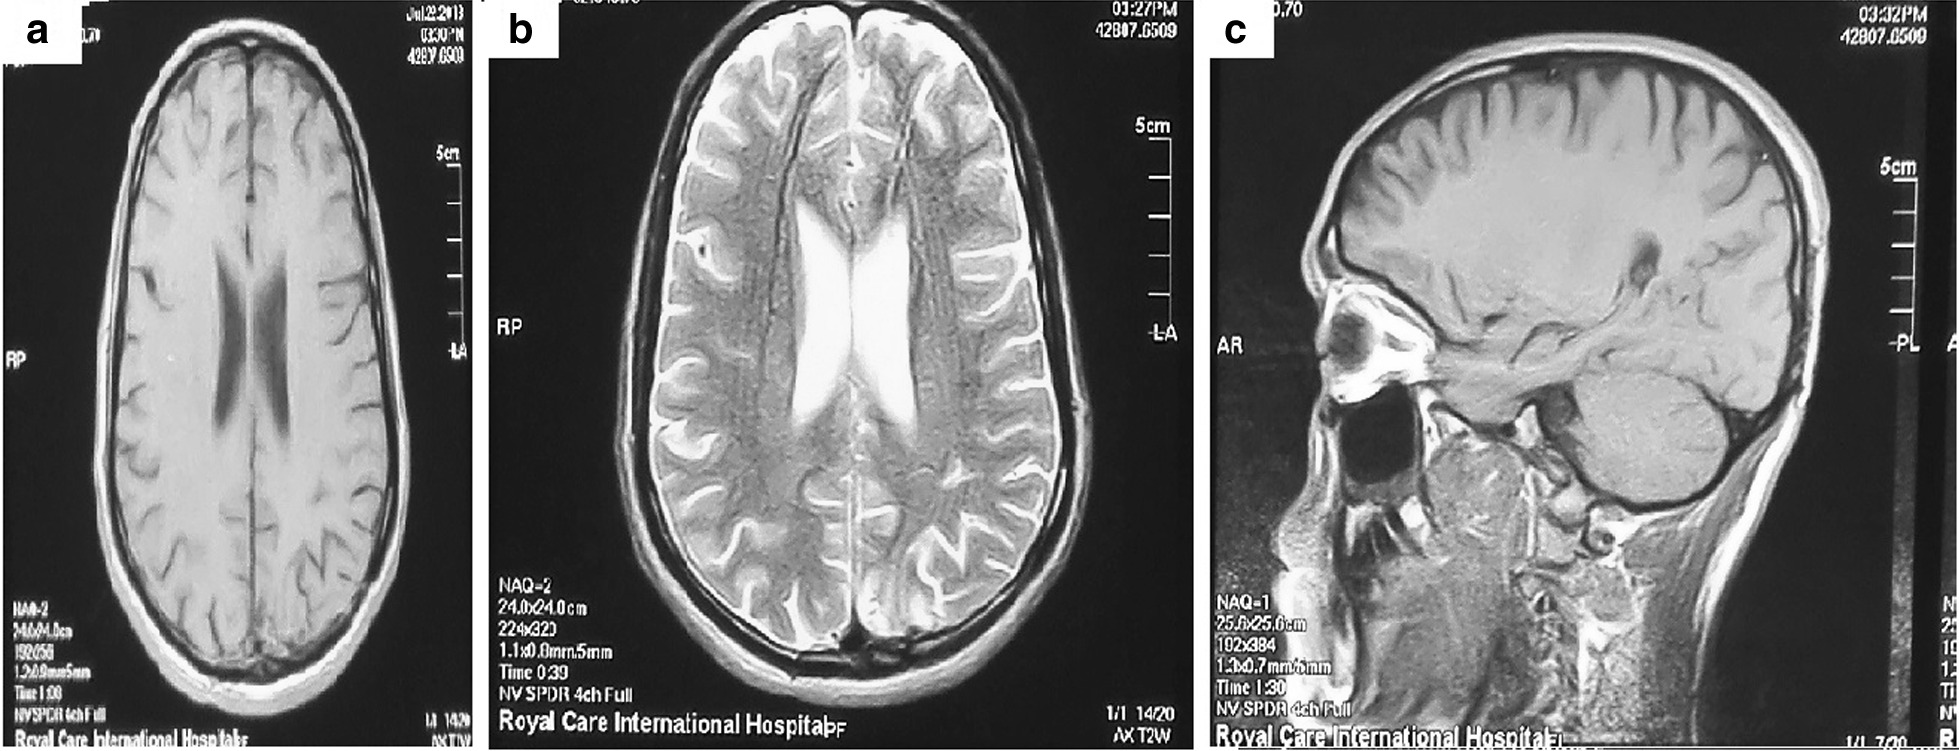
Post-operative MRI brain showed complete resection of the lesion in a) axial T1WI, b) axial T2WI showed left posterior parietal area of gliosis, c) Sagittal T1WI
